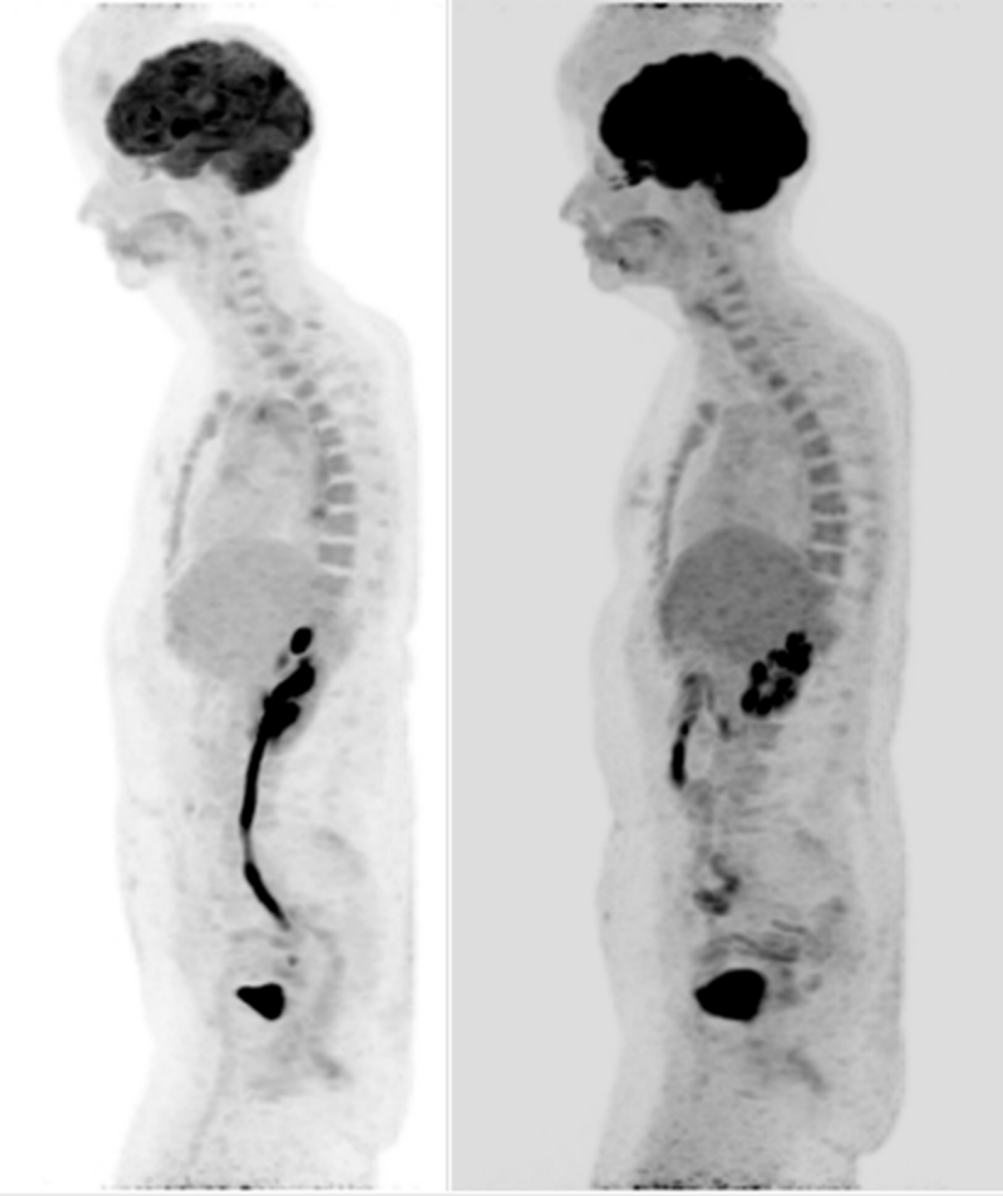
(Left image) MIP sagittal ^18^F-FDG PET-CT shows increased FDG
